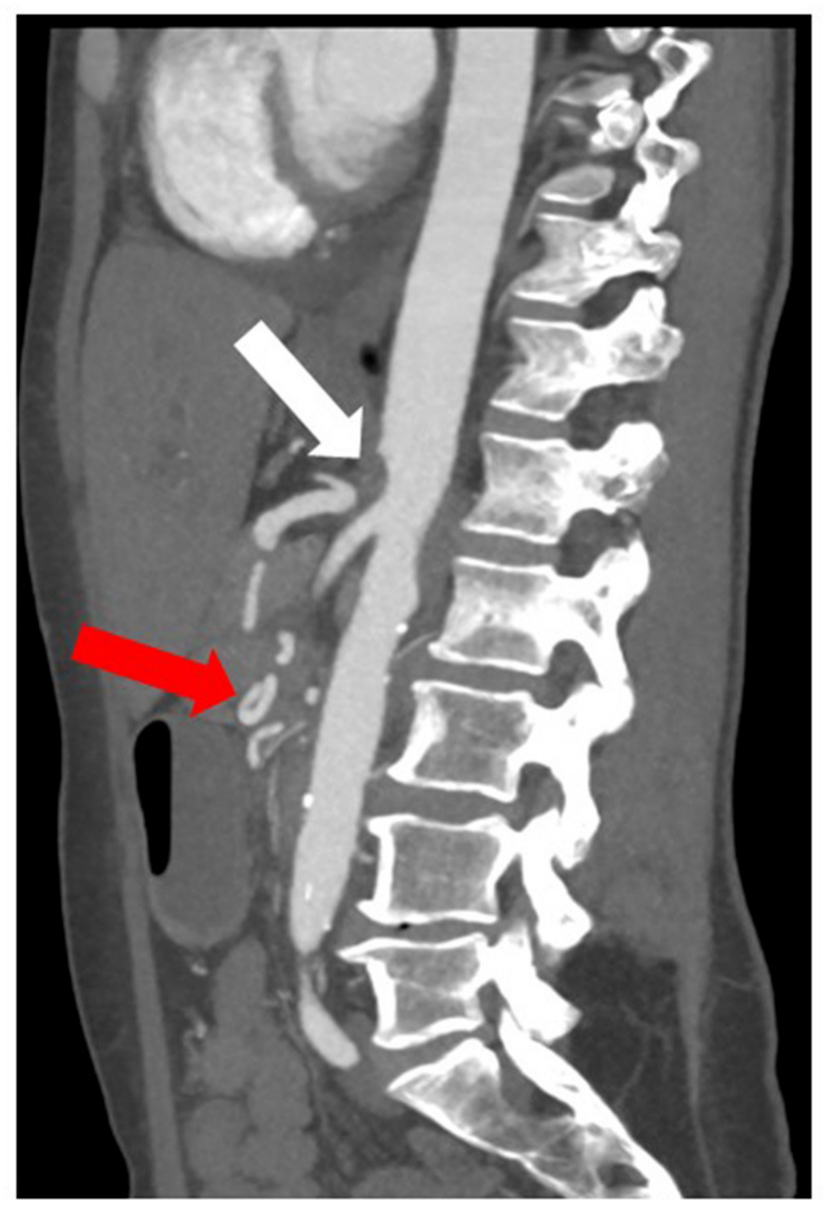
CT image of an anatomic variation of the visceral arteries with aplasia of the origin of the celiac trunk in the aorta (white arrow) and collateralization of the hepatic artery *via* a network of pancreatoduodenal collaterals (red arrow).
